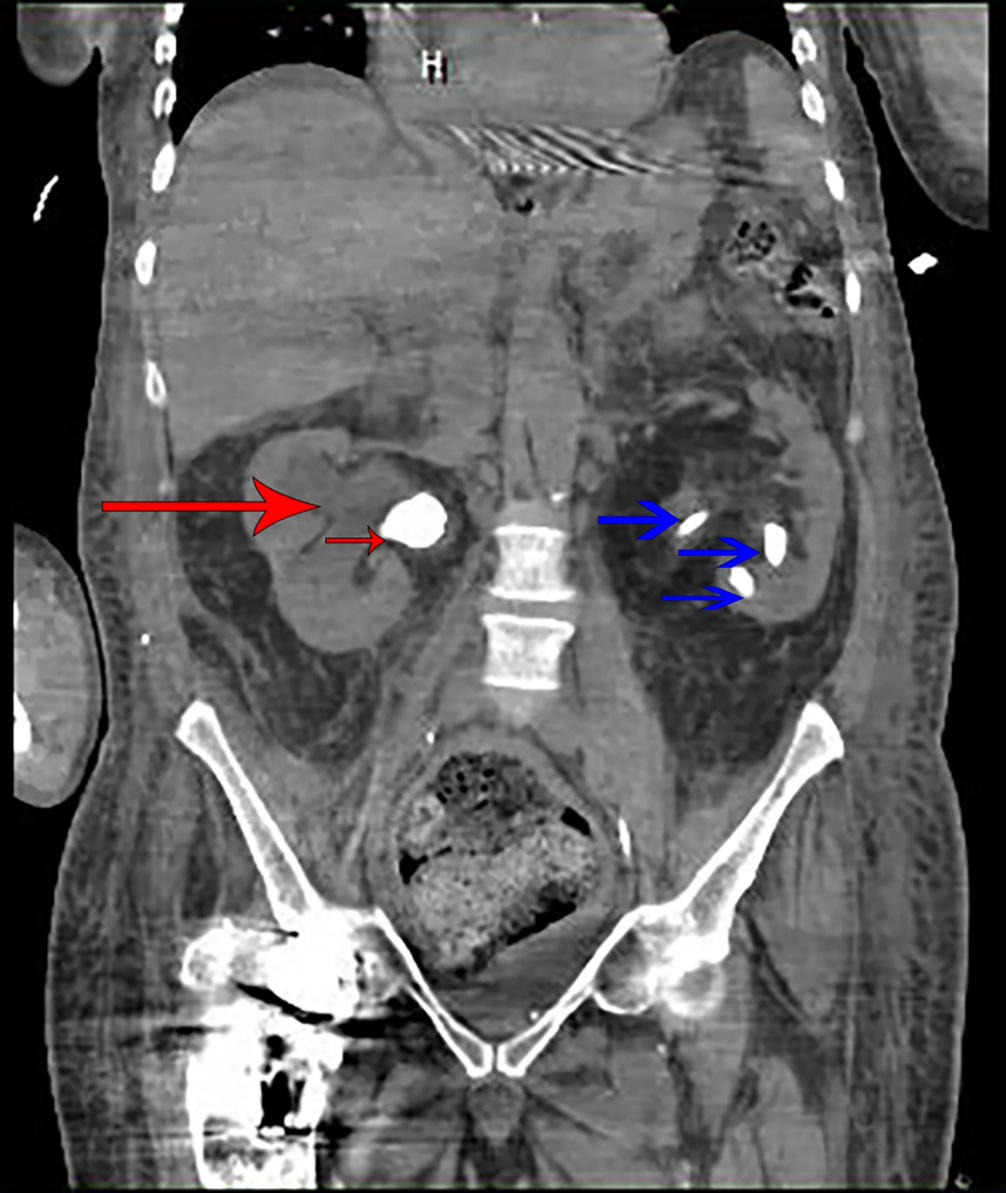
Computed tomography urogram shows moderate right-sided hydronephrosis (large red arrow), one of the staghorn calculi in the right kidney (small red arrow) and three staghorn calculi within the left kidney (three blue arrows)
